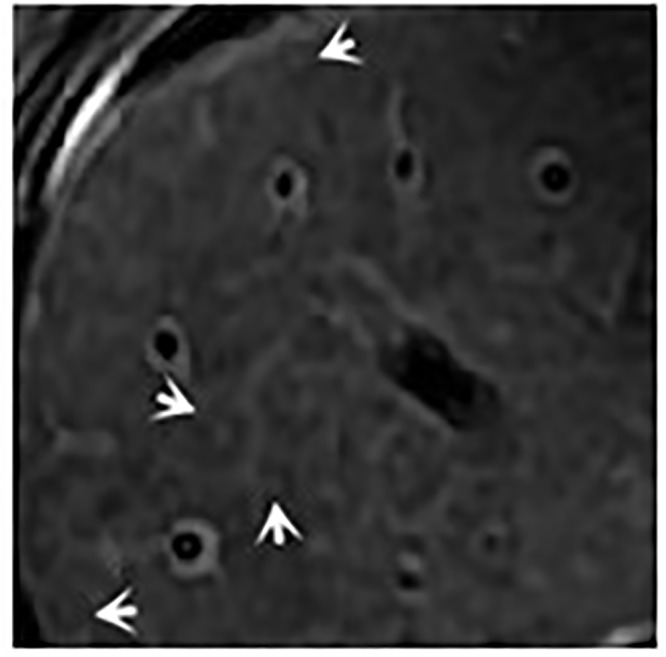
male, 61 years, axial T2-weighted image shows periportal halo signs as hypointense areas around the portal veins (*arrows*).
